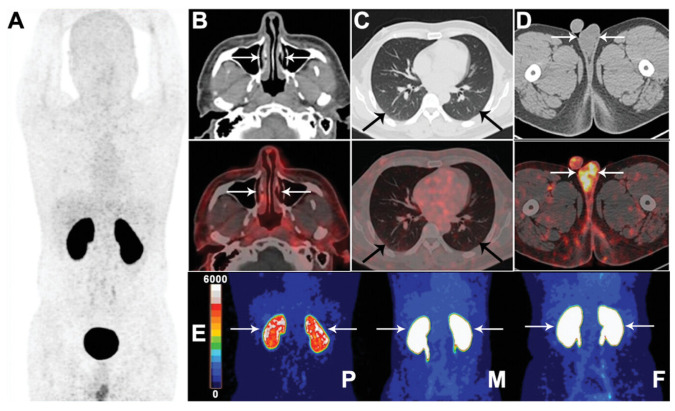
Representative positron emission tomography (PET) images of ^68^Ga-HZ20 in a volunteer recovered from COVID-19 infection. (A) The whole-body PET image and (B–D) axial PET/computed tomography (CT) (top panel: CT, middle panel: PET) images of nasal, lung, and testis, respectively, at 90 min post-injection were shown. (E) kidney uptake of ^68^Ga-HZ20 in a patient recovered from COVID (P), a healthy male (M), and a female (F) volunteer at 90 min post-injection. Arrows indicate regions of interest in (B–E). Image from Zhu, H.; Zhang, H.; Zhou, N.; Ding, J.; Jiang, J.; Liu, T.; Liu, Z.; Wang, F.; Zhang, Q.; Zhang, Z.; et al. Molecular PET/CT profiling of ACE2 expression in vivo: implications for infection and outcome from SARS-CoV-2. Adv. Sci. 2021, 8, e2100965 is licensed under CC BY 4.0 is licensed under CC BY 4.0 (http://creativecommons.org/licenses/by/4.0) [93].
